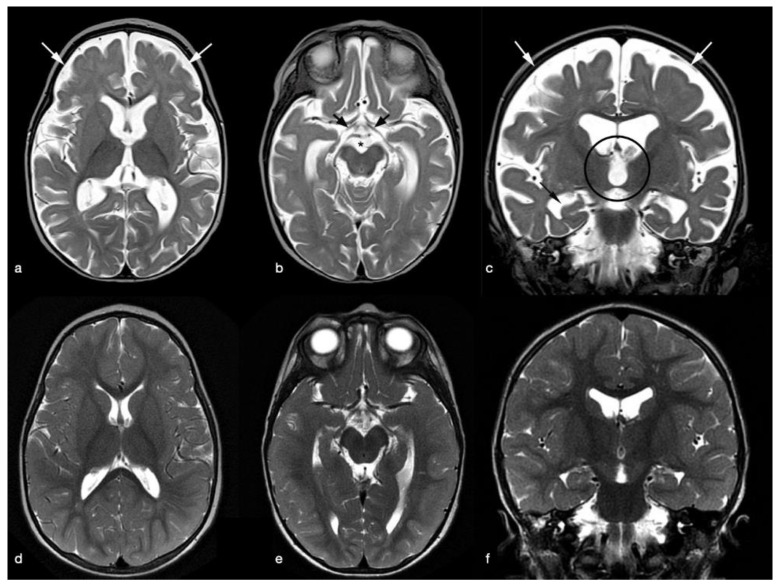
MRI exams performed (a–c) at admission to hospital and (d–f) 10 months after the start of Vitamin B12 treatment. (a,b) Axial (d,e) and coronal (c,f) T2-weighted images. There is evidence of a mild cerebral atrophy characterized by an enlargement of cortical sulci and subarachnoid spaces (white arrows in (a,c)). Enlargement of the lateral and third ventricles (open circle and black arrow in (c)), and the perimesenchephalic cistern (asterisk in (b)). Thinning of the post-chiasmatic optic nerves (black arrows in (b)). (d–f) Disappearance of all structural alterations after therapy.
